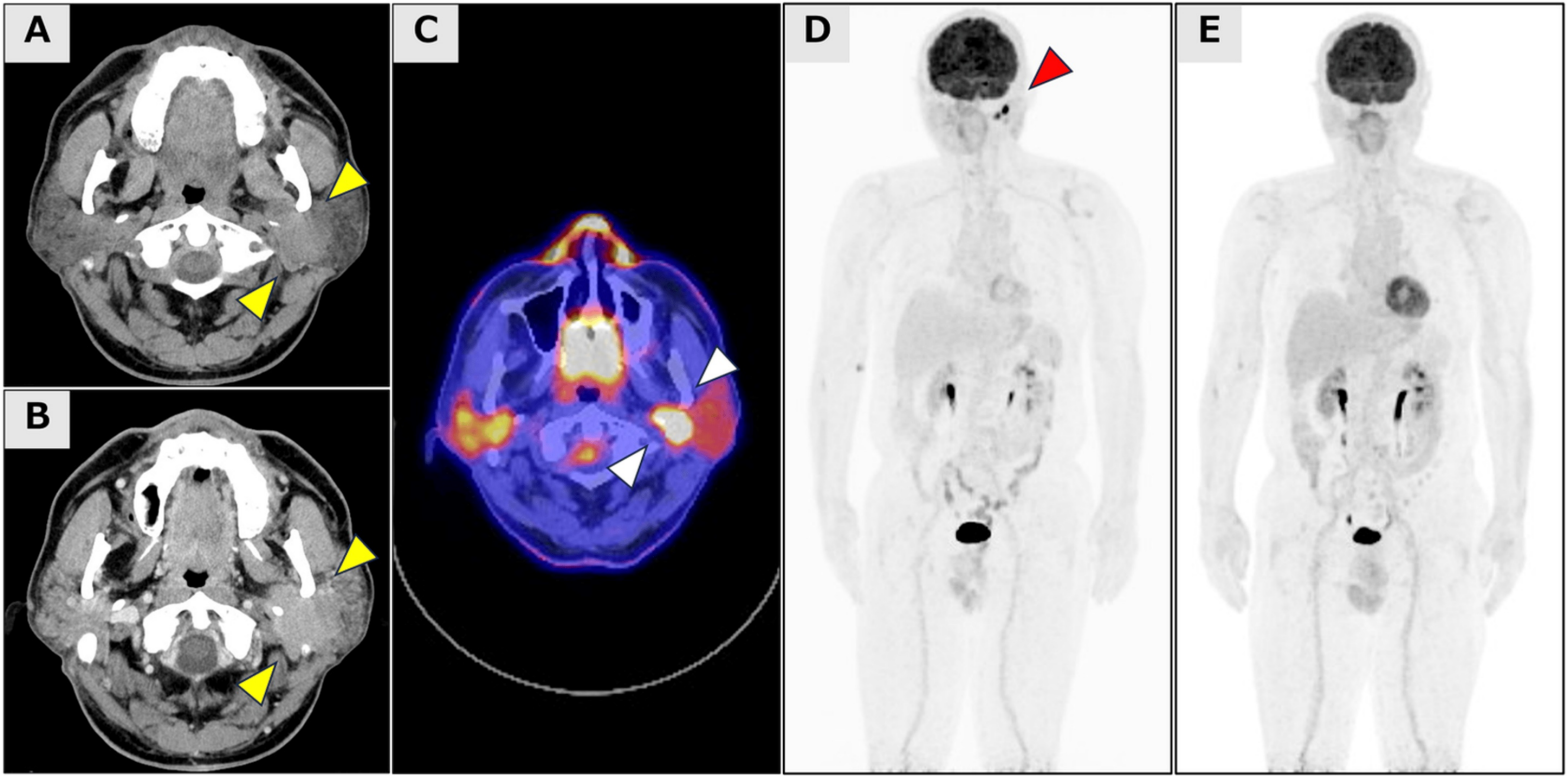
Radiological findings at baseline and post-treatment Axial plain (A) and contrast-enhanced (B) computed tomography (CT) images demonstrate the left parotid tumor with a maximum diameter of approximately 3 cm in the greatest dimension. (yellow arrow) 18-fluorodeoxyglucose positron emission tomography/CT (FDG-PET/CT) demonstrates intense hypermetabolic activity (SUVmax 38.29) corresponding to the left parotid gland lesion (white arrow). (C) Maximum intensity projection (MIP) images of FDG-PET obtained at initial diagnosis (D) and subsequent to the completion of three cycles of R-CHOP therapy (E) demonstrate complete resolution of metabolic activity in the parotid gland lesion (red arrow).
